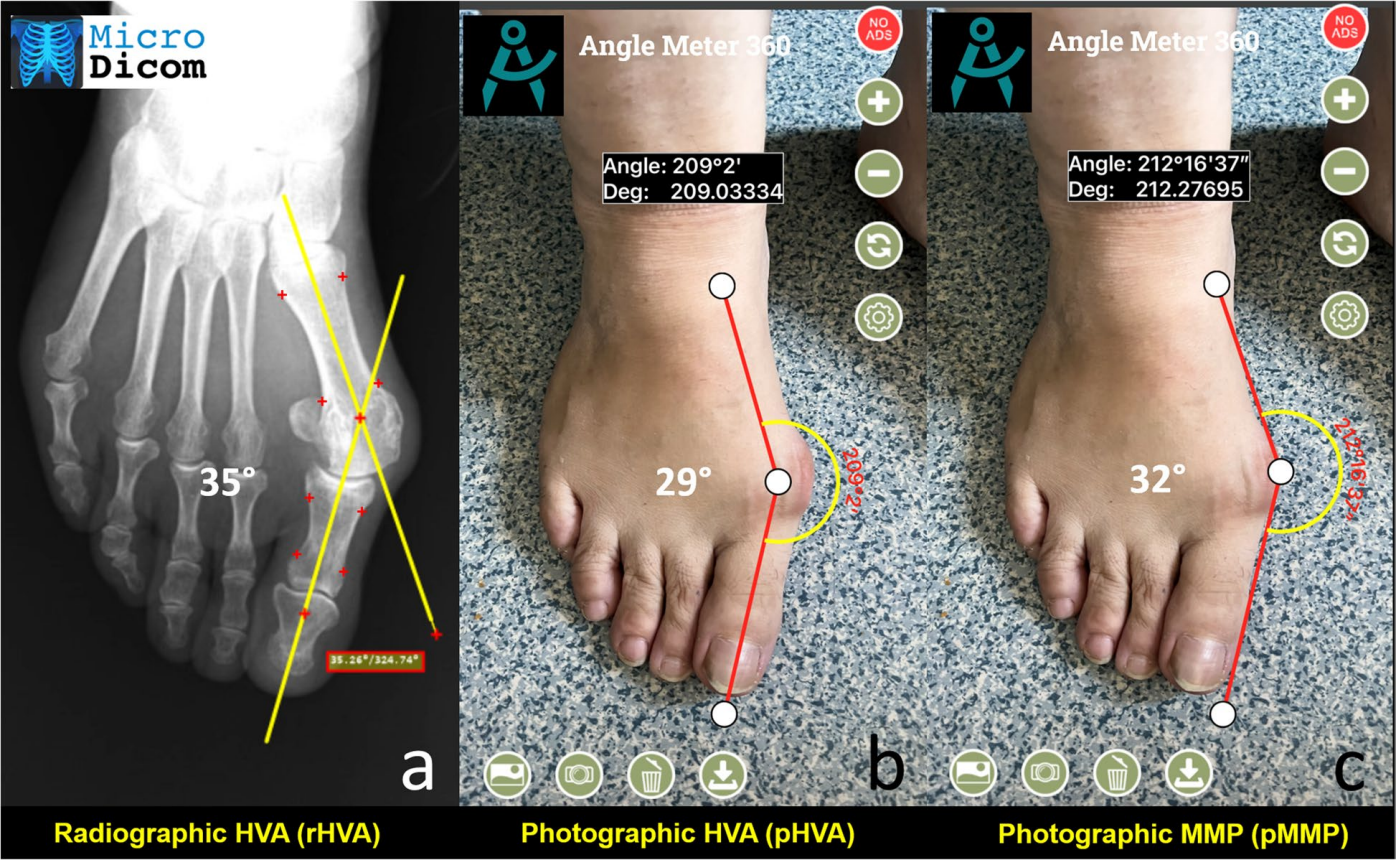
a Radiographic HVA measurement. b Photographic HVA (pHVA) measurement. c Photographic margo medialis pedis (pMMP) measurement
